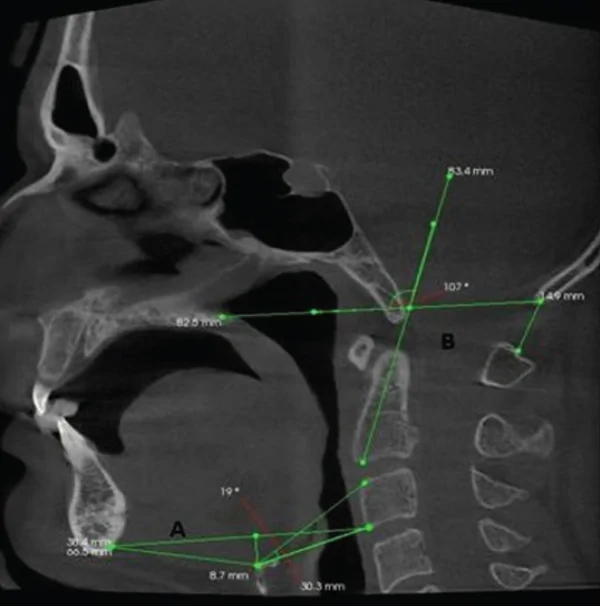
Posttreatment CBCT of Case 1 reveals the hyoid bone at the level of C3 vertebrae. (A) An upward‐facing triangle with an angulation of 19 degrees. (B) Cervical vertebrae alignment.
